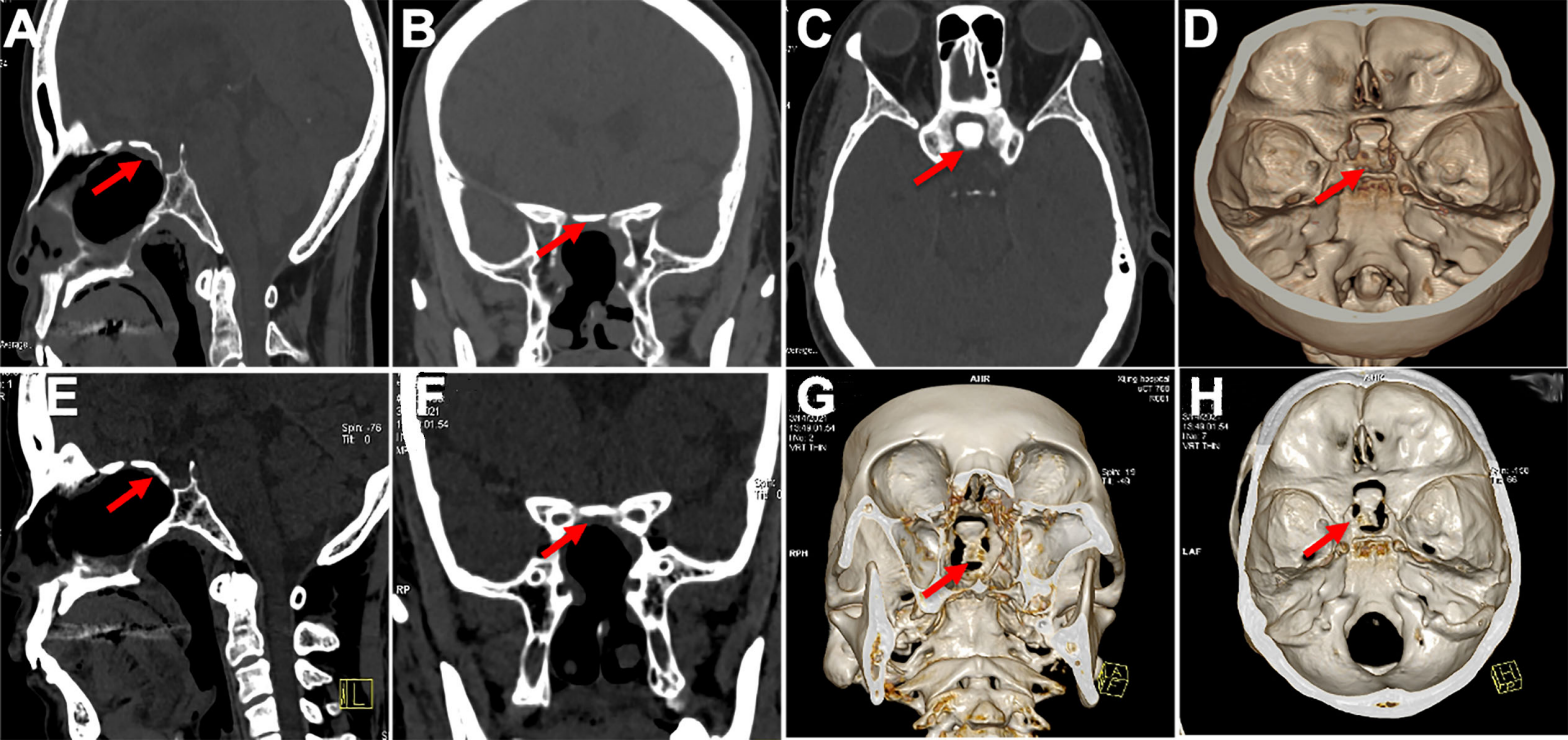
Displayed in situ bone flap postoperatively in 3D reconstruction. (A–D) In situ bone flaps were in good position and achieved complete osseous reconstruction 6 months after surgery. (E–H) The bone flap was not absorbed, and there was new ossification between the bone flap and surroundings on CT images 18 months after surgery.
